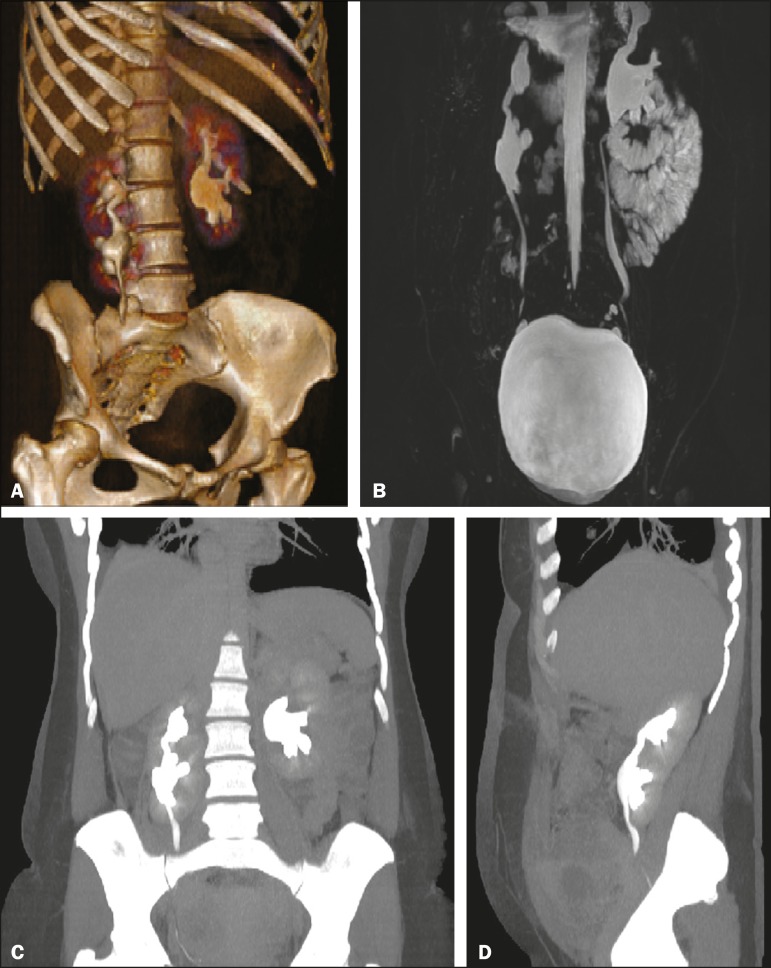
A: 3D reconstruction of a CT scan of the abdomen (excretory
